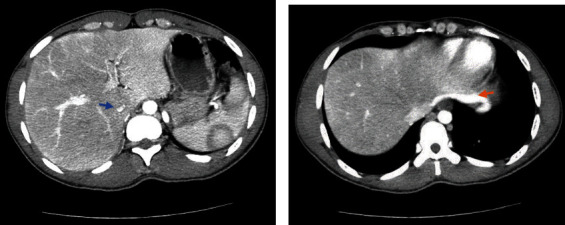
(a) CT abdomen/pelvis with contrast showing intrahepatic IVC stenosis (blue arrow). (b) Seen is a large diaphragmatic venous collateral to the IVC (orange arrow), indicating that the thrombosis in this patient was acute on chronic pathology.
